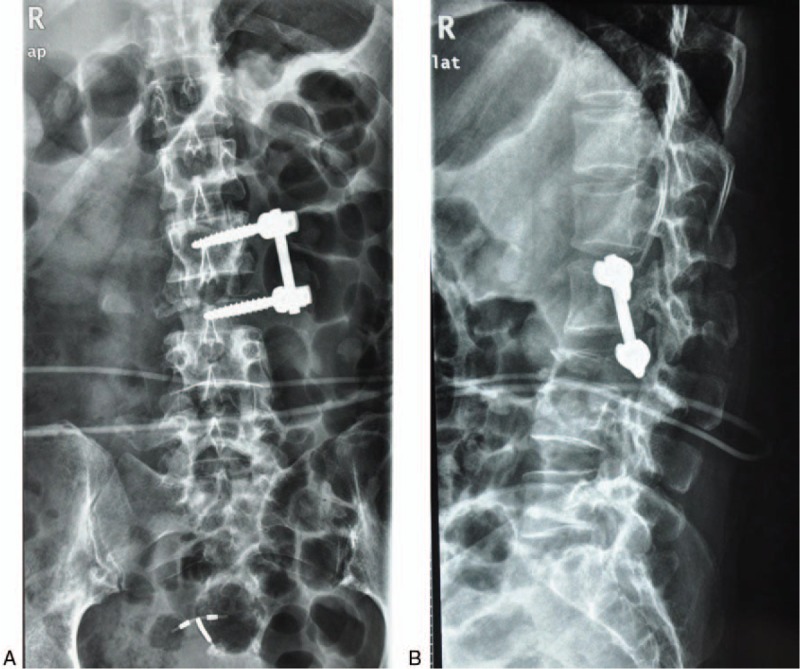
X-rays of dorsal spine revealed disappearance of lumbar physiological curvature, half-baked L2–L3 centrums, fixation in L2–L3, and swelling paravertebral soft tissue.
